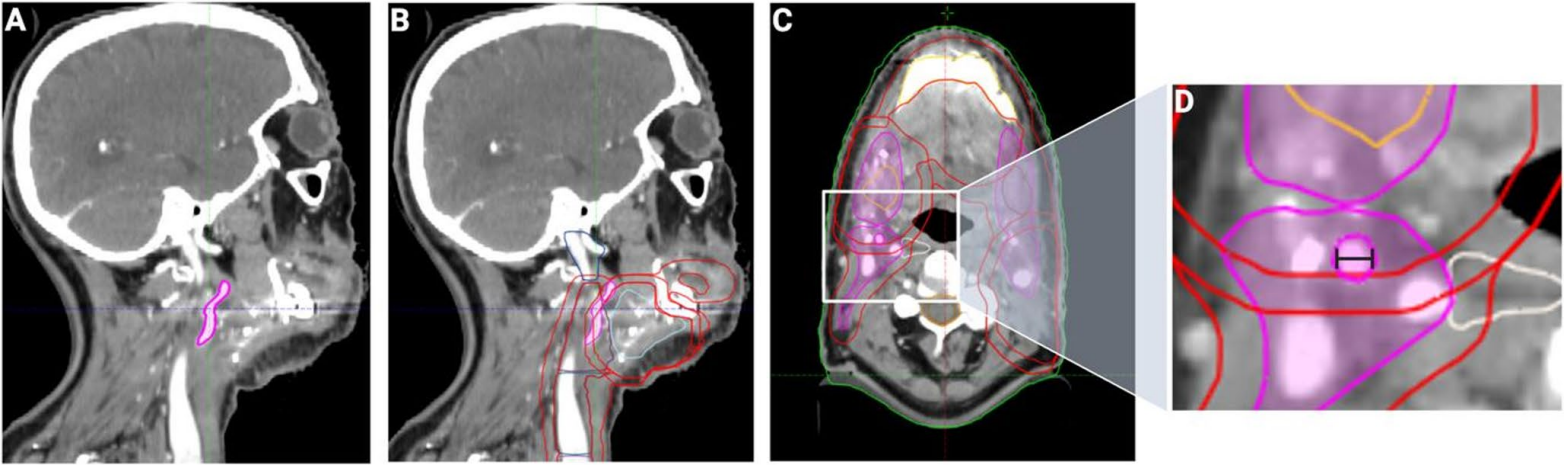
Exemplary depiction of the external carotid artery (ECA). The delineated ECA (magenta) is depicted in sagittal view of the planning computed tomography (CT) without (A) and with (B) corresponding radiation volumes. (C) shows the axial view of the radiation volumes with the ECA. Inset (D) presents an exemplary measurement of the ECA diameter (black line)
